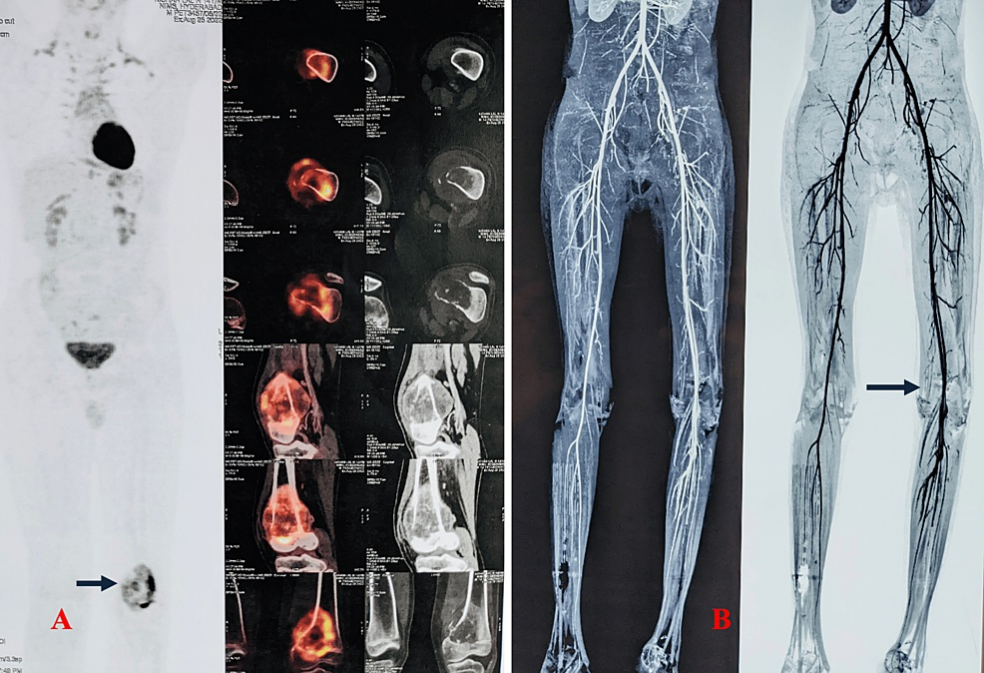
Pre-biopsy imaging evaluation: PET-CT and CT angiography. A: Whole-body PET-CT to rule out metastasis. B: CT angiography of the involved limb. PET-CT = positron emission tomography-computed tomography
